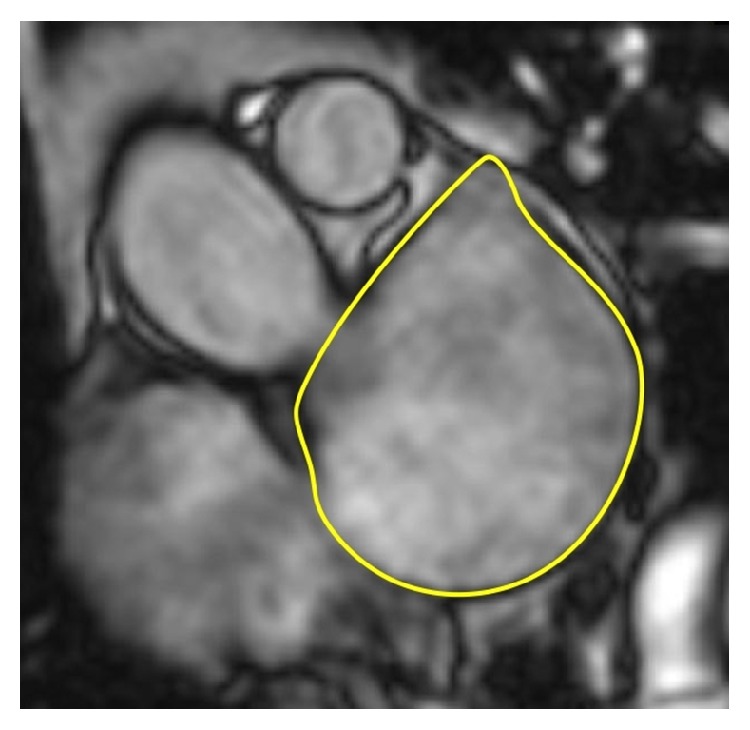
Representative slice demonstrating the tracing of the left atrial boundary at ventricular end-systole in short-axis view on steady-state free precession magnetic resonance image. The left atrial volume is then calculated using the disk area summation method (Simpson's method) from contiguous slices covering the whole left atrial cavity.
